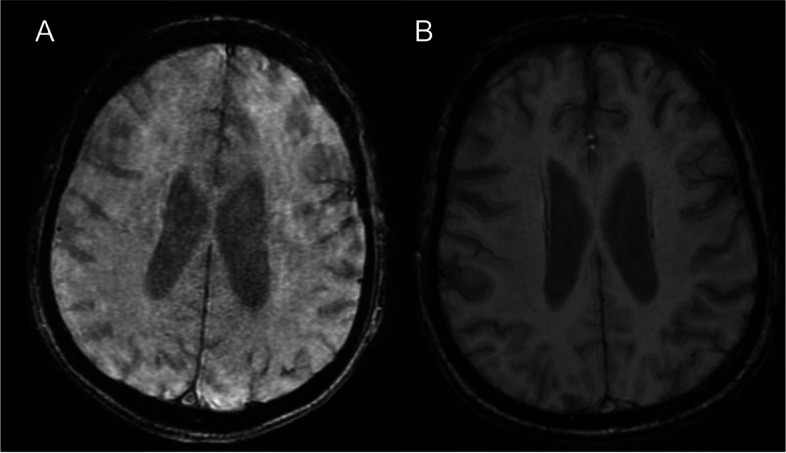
Comparison between SWAN MRI sequences before and after early neurological deterioration. A Day 0 SWAN MRI before early neurological deterioration. B Day 1 SWAN MRI after early neurological deterioration
